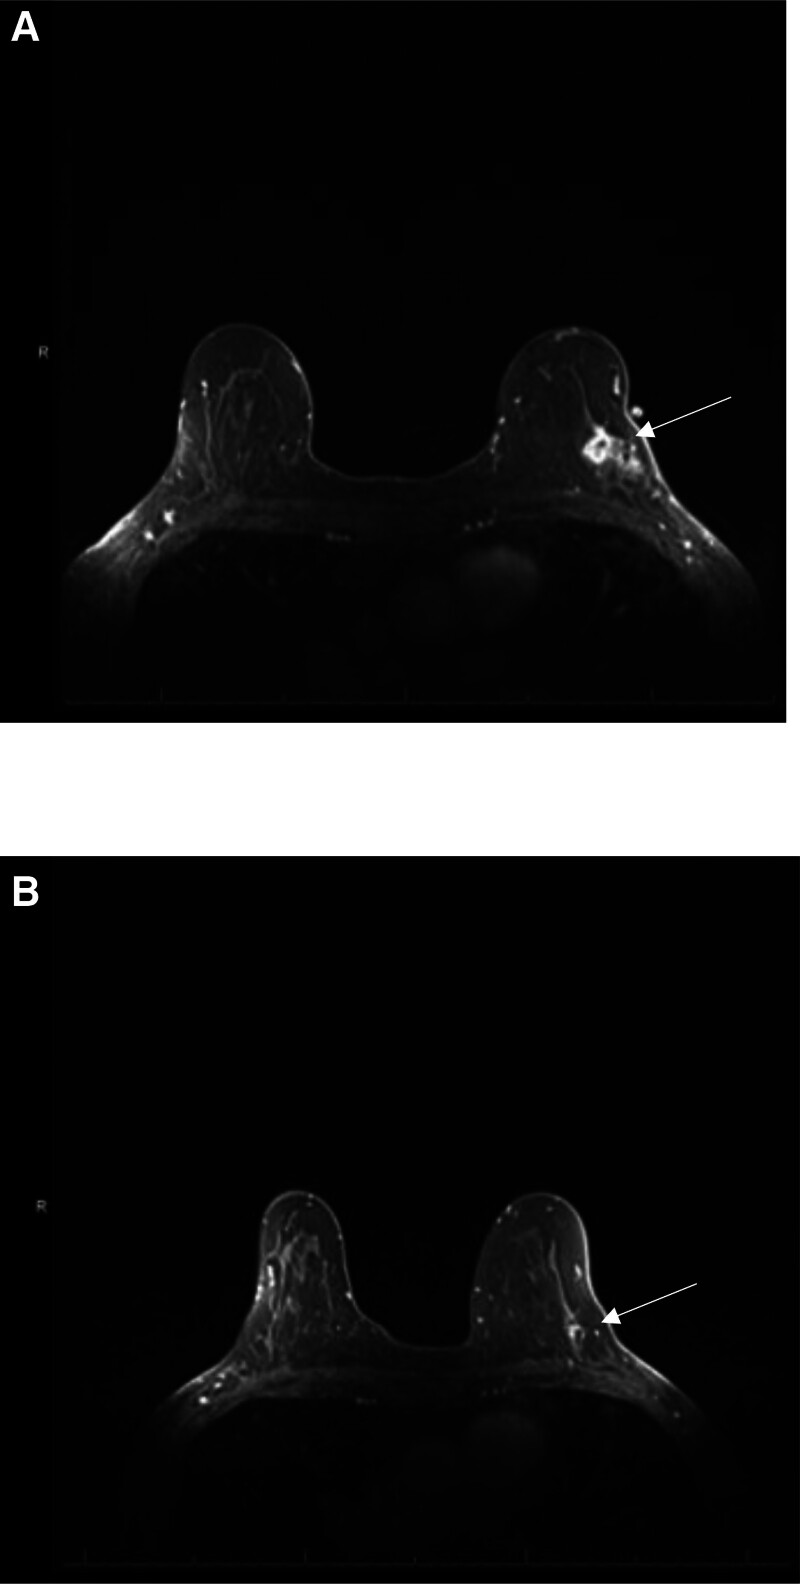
Axial T1-weighted post-gadolinium breast magnetic resonance imaging (MRI) of Case 2. (A) MRI before treatment showing a lobulated enhancing mass (arrow); (B) MRI after treatment showing resolution of the enhancing mass (arrow). MRI = magnetic resonance imaging.
